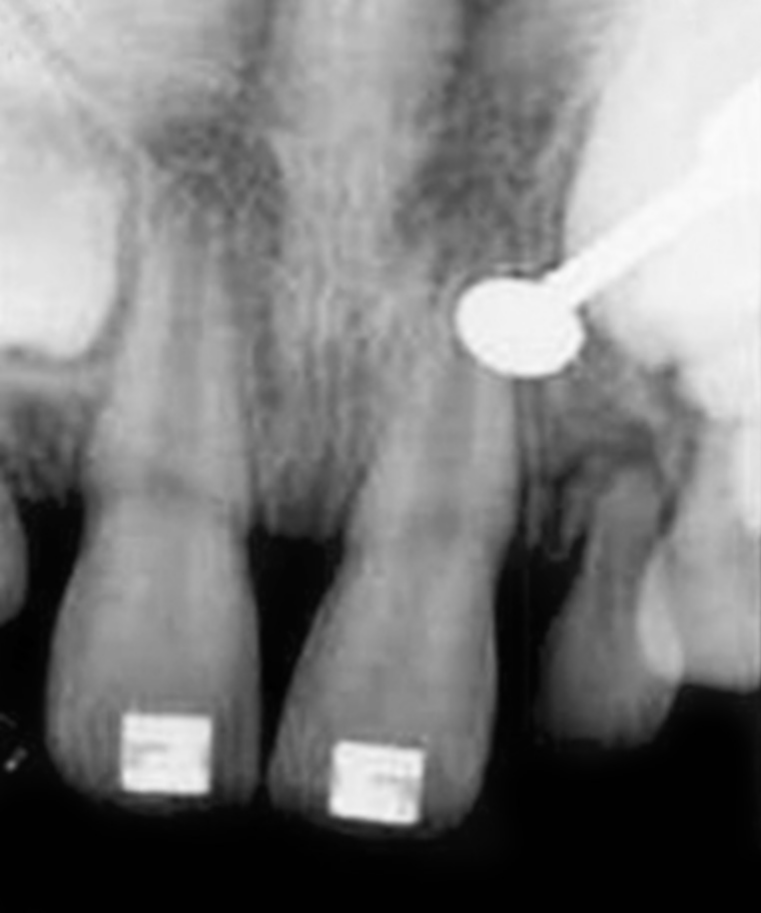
Post-treatment periapical radiograph showing closure of midline diastema
